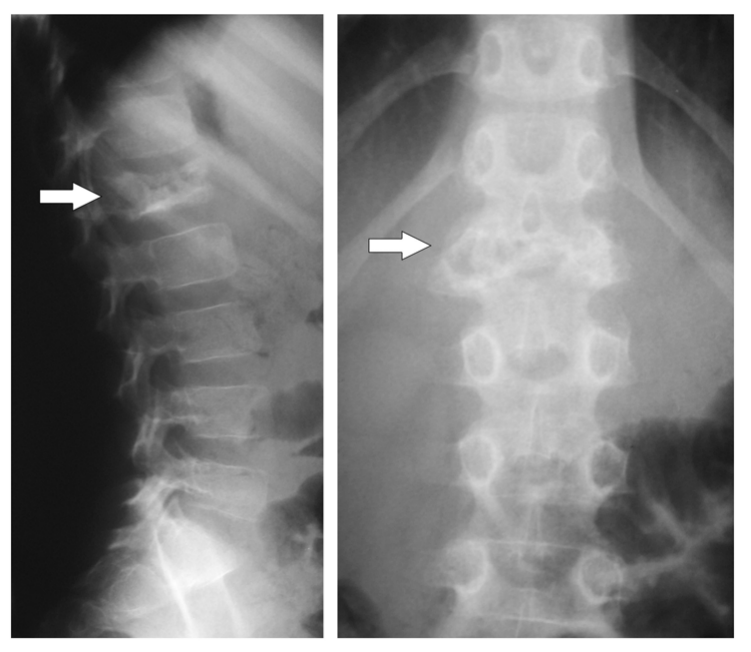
Plain radiographs (AP and Lateral) of lumbar spine. Demonstrate destruction of the L1 vertebra (arrow).
